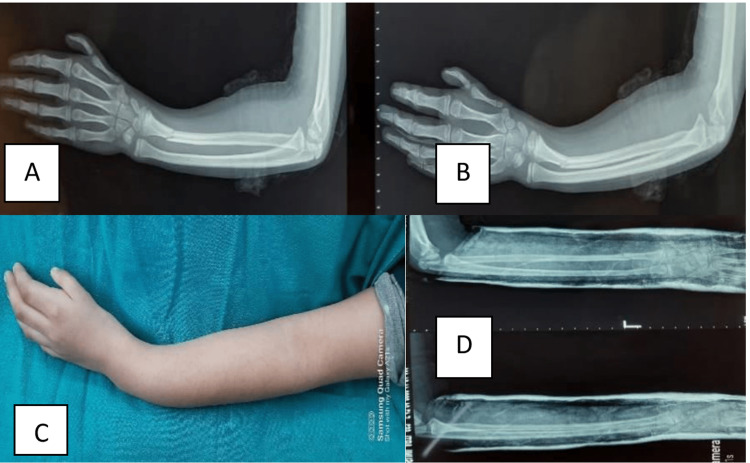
Pre-reduction and post-reduction X-rays of forearm fracture (A, B) Pre-reduction X-ray of forearm showing greenstick fracture distal radius. (C) Clinical picture showing deformity at wrist. (D) Post-reduction X-ray showing satisfactory alignment.
